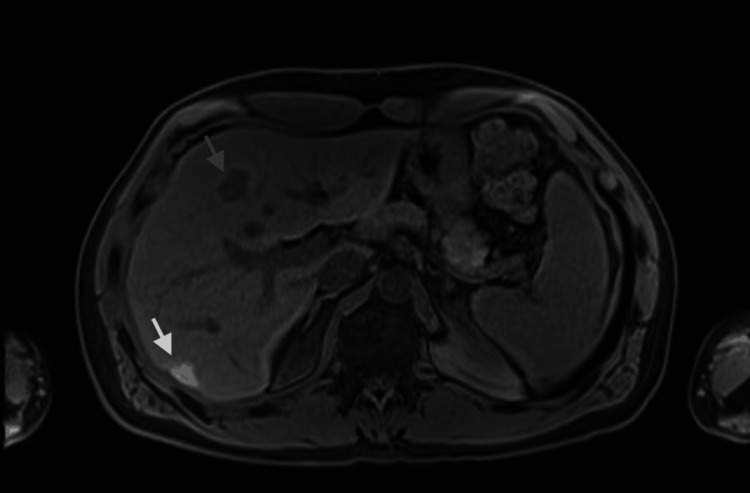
Follow-up MRI axial plane Gray arrow: Metastasis at the interface of segments 8/4a with a reduction in size from 40 to 26 mm. White arrow: In the posterior segment, at the site where a secondary lesion was previously visualized, there is a focus of T1 hyperintensity of 18 mm, secondary to coagulative necrosis transformation post-RFA. No foci of tumor viability were detected within it. MRI: magnetic resonance imaging, RFA: radiofrequency ablation
